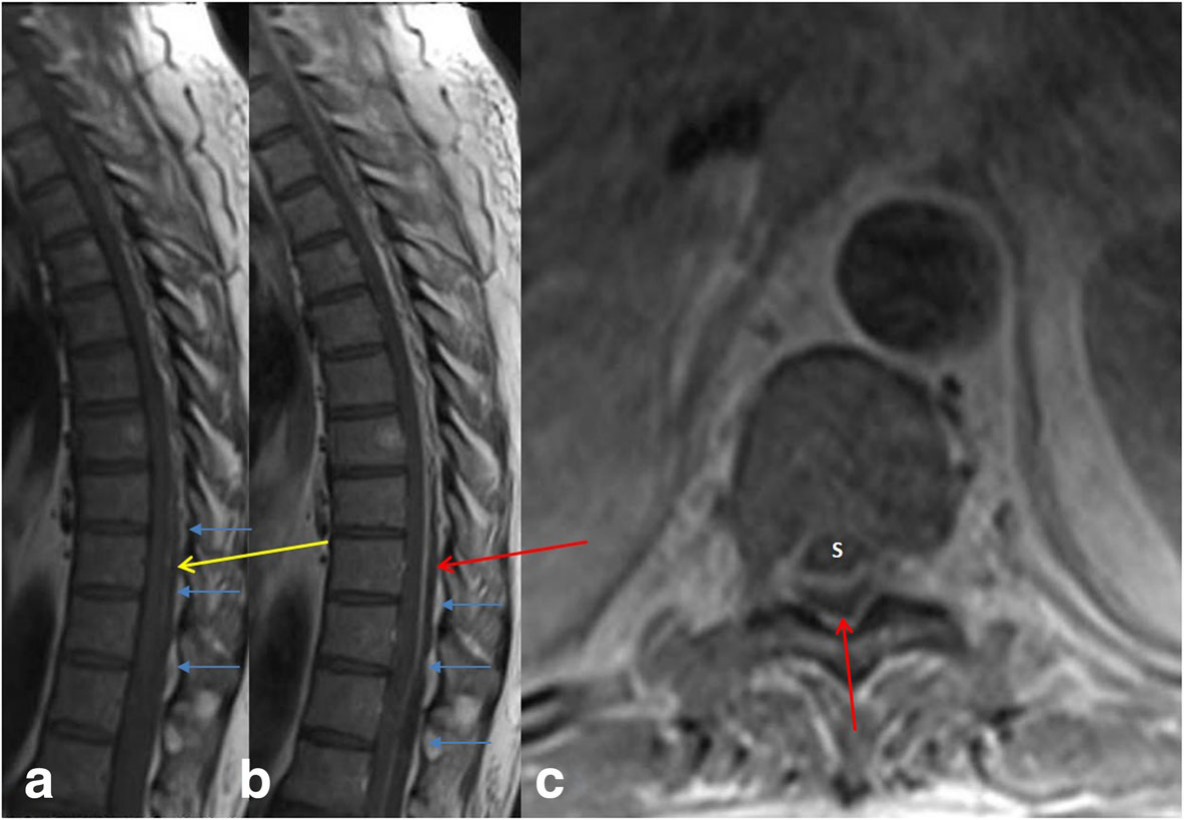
MR imaging in a 24-year-old intravenous drug abuser male shows heterogeneous signal in the subdural space in the lower thoracic spine (*yellow arrow* in a) on T1 W images. There is peripheral rim enhancement on post contrast T1 W images (*red arrows* in b and c), consistent with subdural abscess causing anterior displacement of the cord (marked as S in c). Also note the preserved epidural fat suggesting subdural location of the abscess (*blue* arrows in a and b). Biopsy of the abscess confirmed *Pseudomonas aeruginosa* infection
